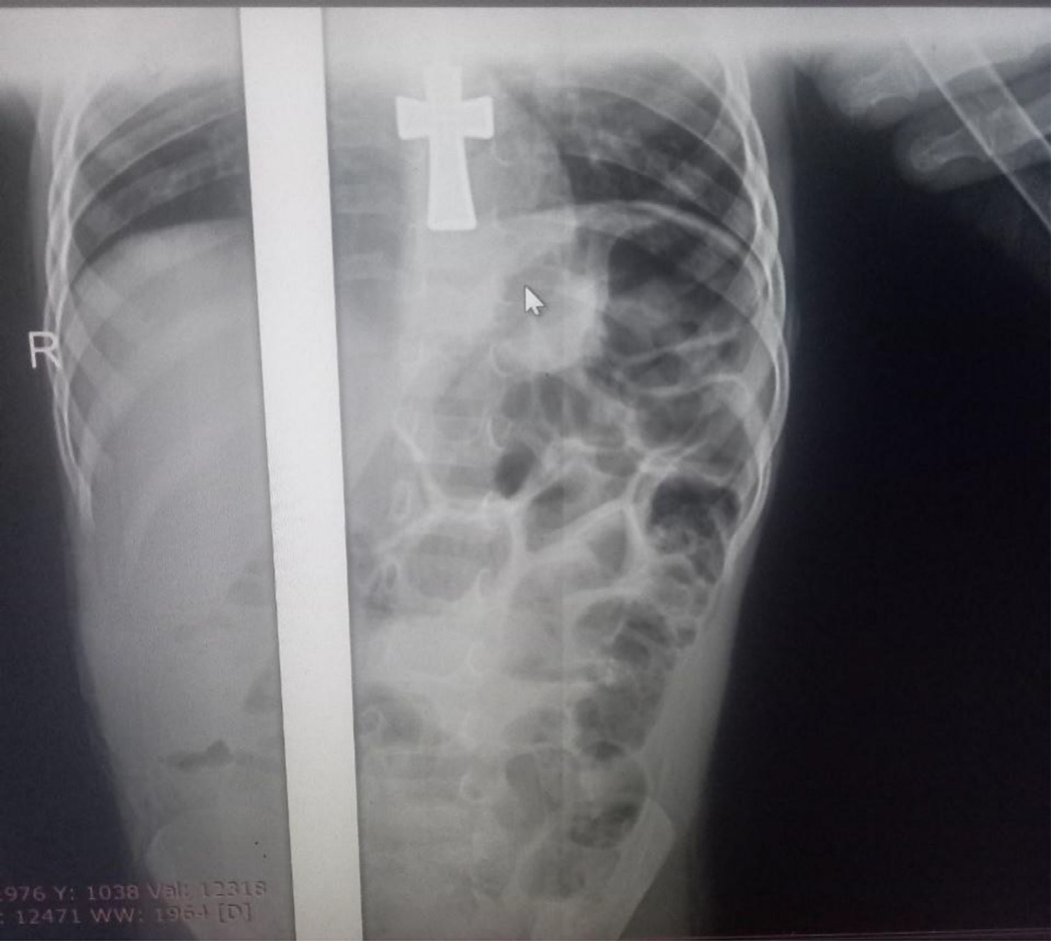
Postoperative X-ray reveals unremarkable finding with no air fluid level or distended bowel loop.
